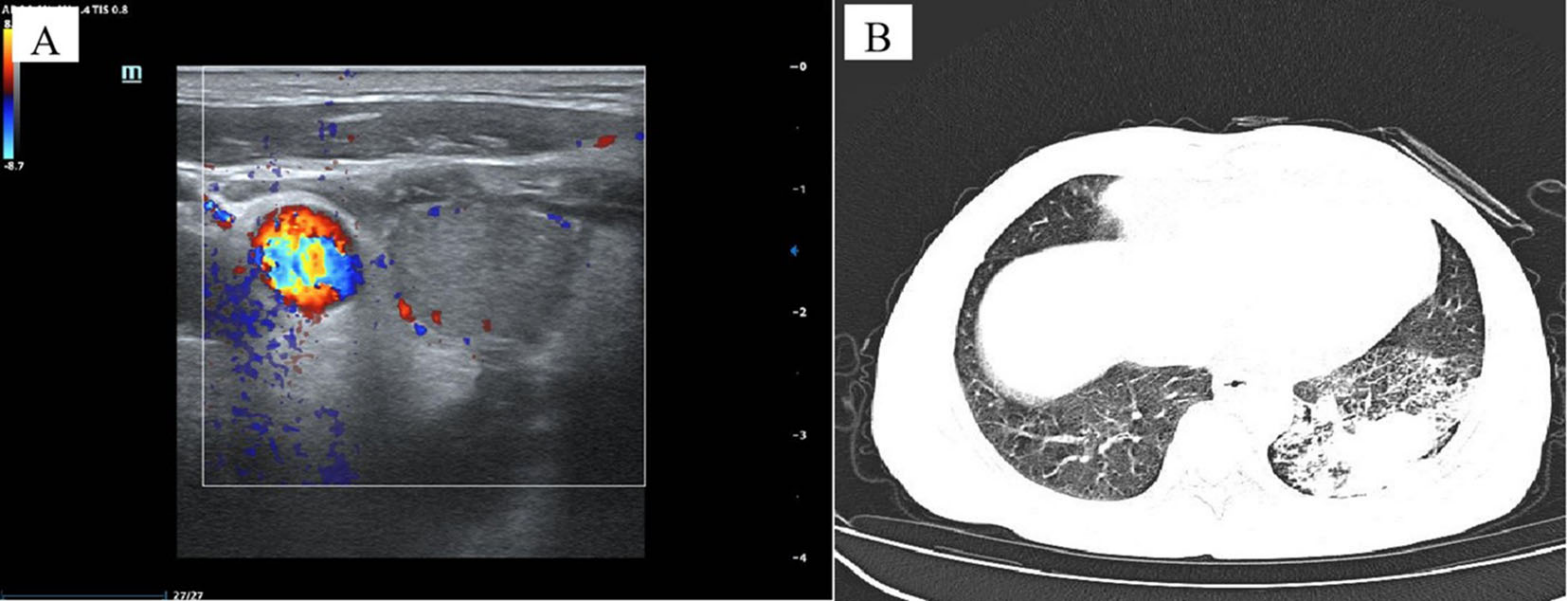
Imaging data of the case. Ultrasonography of the right thyroid lobe showed an irregular, hypoechoic nodule. Doppler ultrasound showed intra-nodular low vascularization (A). Chest Computed Tomography (CT) scan showed patchy soft tissue density shadows in the left lower lung, resembling pneumonia (B).
